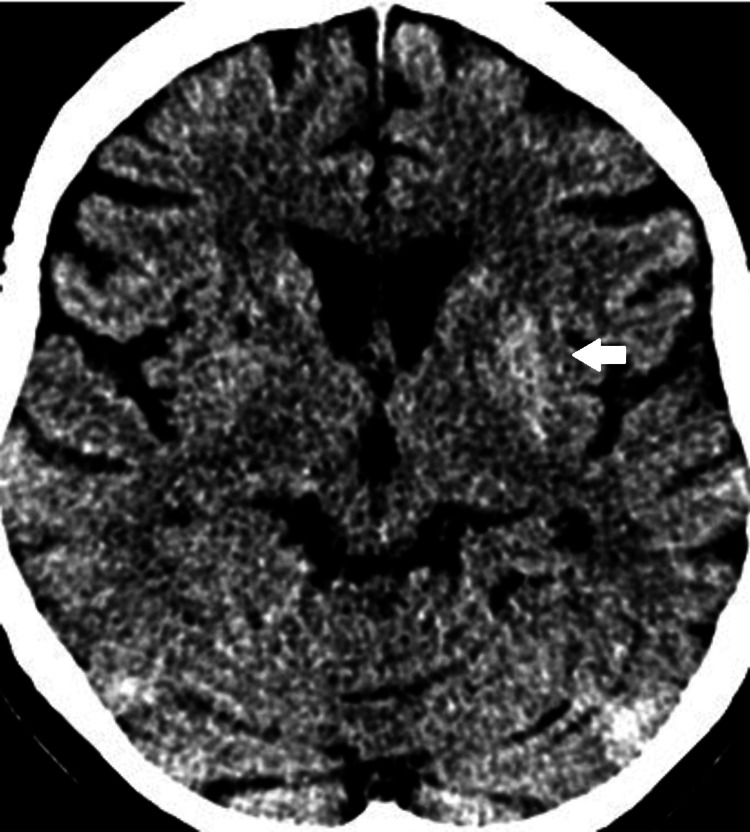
Non-contrasted CT brain showed residual faint hyperdensity of the left basal ganglia
